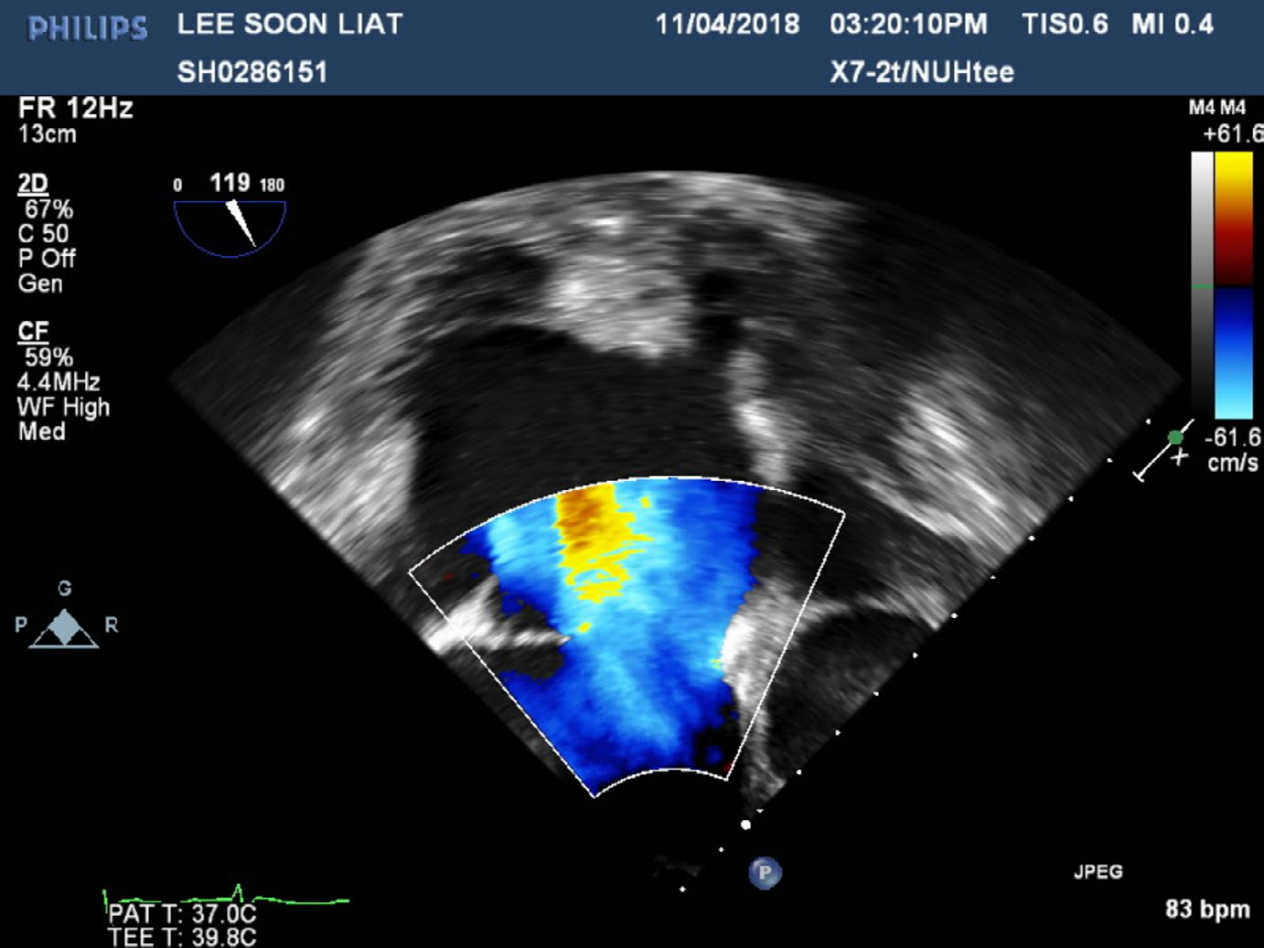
Preoperative Transesophageal Echocardiogram showing color flow across ASD
